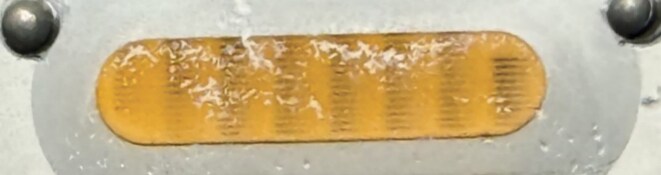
Radiation-induced color centers in a 1 wt % sample of GdPO_4_.0.5H_2_O in boron nitride following Gd Lα_1_ RIXS measurements at cryogenic temperature. The dark horizontal streaks in the sample correspond to regions exposed to the X-ray beam. The sample window is ∼20 mm wide in this image.
